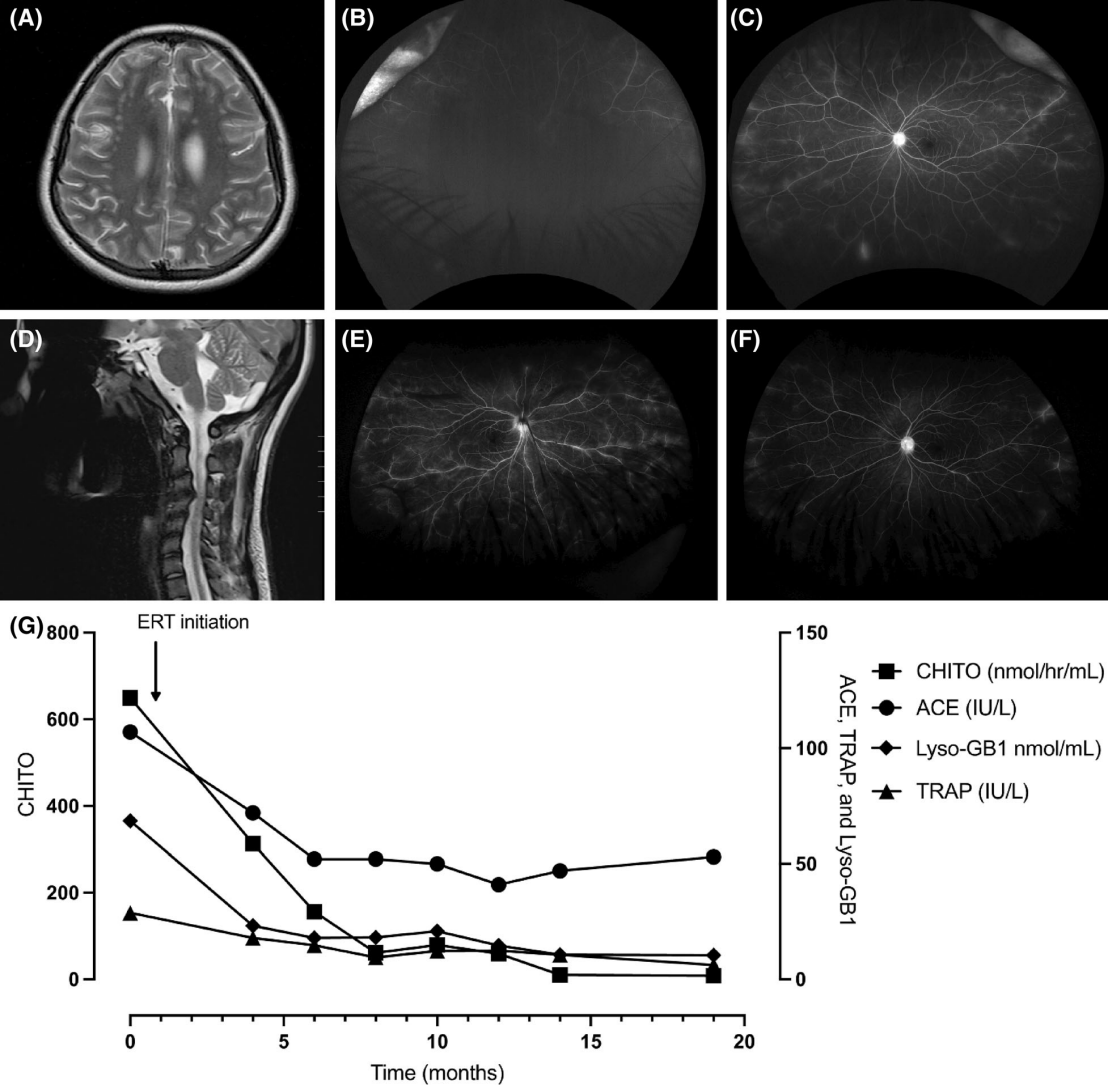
Magnetic resonance imaging (MRI) of brain and spinal cord, fluorescein angiograms, and biomarker evaluations of our patient with Gaucher disease type 3c before and after enzyme replacement therapy initiation. At age 14 years, (A) the brain MRI, T2 revealed multiple nonspecific small foci of white matter T2/FLAIR hyperintensity within right greater than left cerebral hemispheres. Additionally, (B) the right eye details were obscured due to vitreous hemorrhage and (C) the left eye showed mid peripheral and peripheral perivascular leak. At age 17 years, (D) the MRI cervical spine, T2 demonstrated severe spinal canal stenosis with deformation of the spinal cord and cord signal abnormality, suggestive of compressive myelopathy. Enzyme replacement therapy (ERT) was started at age 18 years and by age 20 years, (E) there was complete resolution of the vitreous hemorrhage in the right eye and (F) there was reduced mid peripheral and peripheral perivascular leak in the left eye. Accordingly, (G) the biomarkers chitotriosidase (CHITO), angiotensin‐converting enzyme (ACE), Glucosylsphingosine (Lyso‐GB1), and tartrate resistant acid phosphatase (TRAP) decreased after enzyme replacement therapy initiation.
